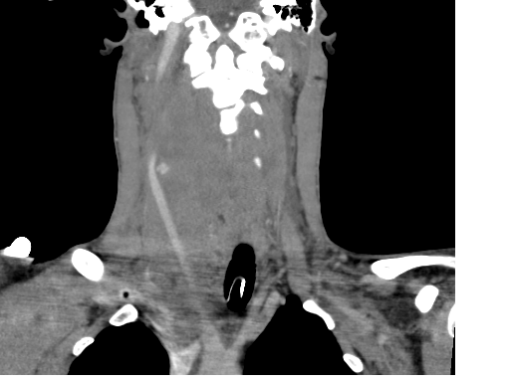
CT angiogram, coronal section showing active leak of contrast from the right common carotid artery. Note the marked displacement of airway to the left.
